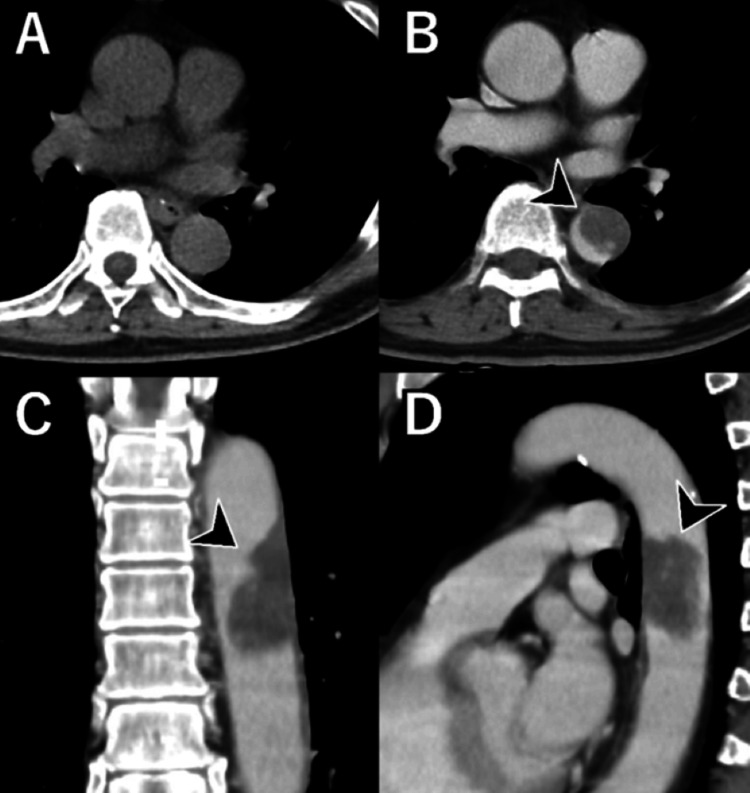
Contrast-enhanced computed tomographic images. On non-contrast CT, the tumor was poorly visualized (A). On contrast-enhanced CT, a 25 mm-diameter, pedunculated tumor protruding into the descending aorta is observed (B, C, D: arrowheads). Contrast enhancement of the lesion was not clearly observed.
